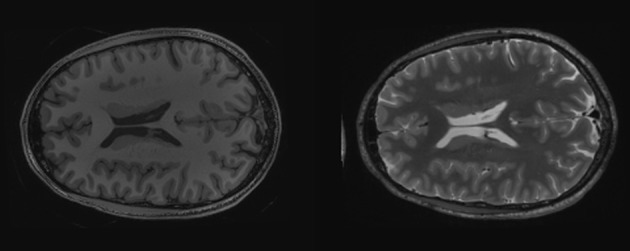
Axial cross sections of T1 (left) and T2 (right) MRI of a brain.
